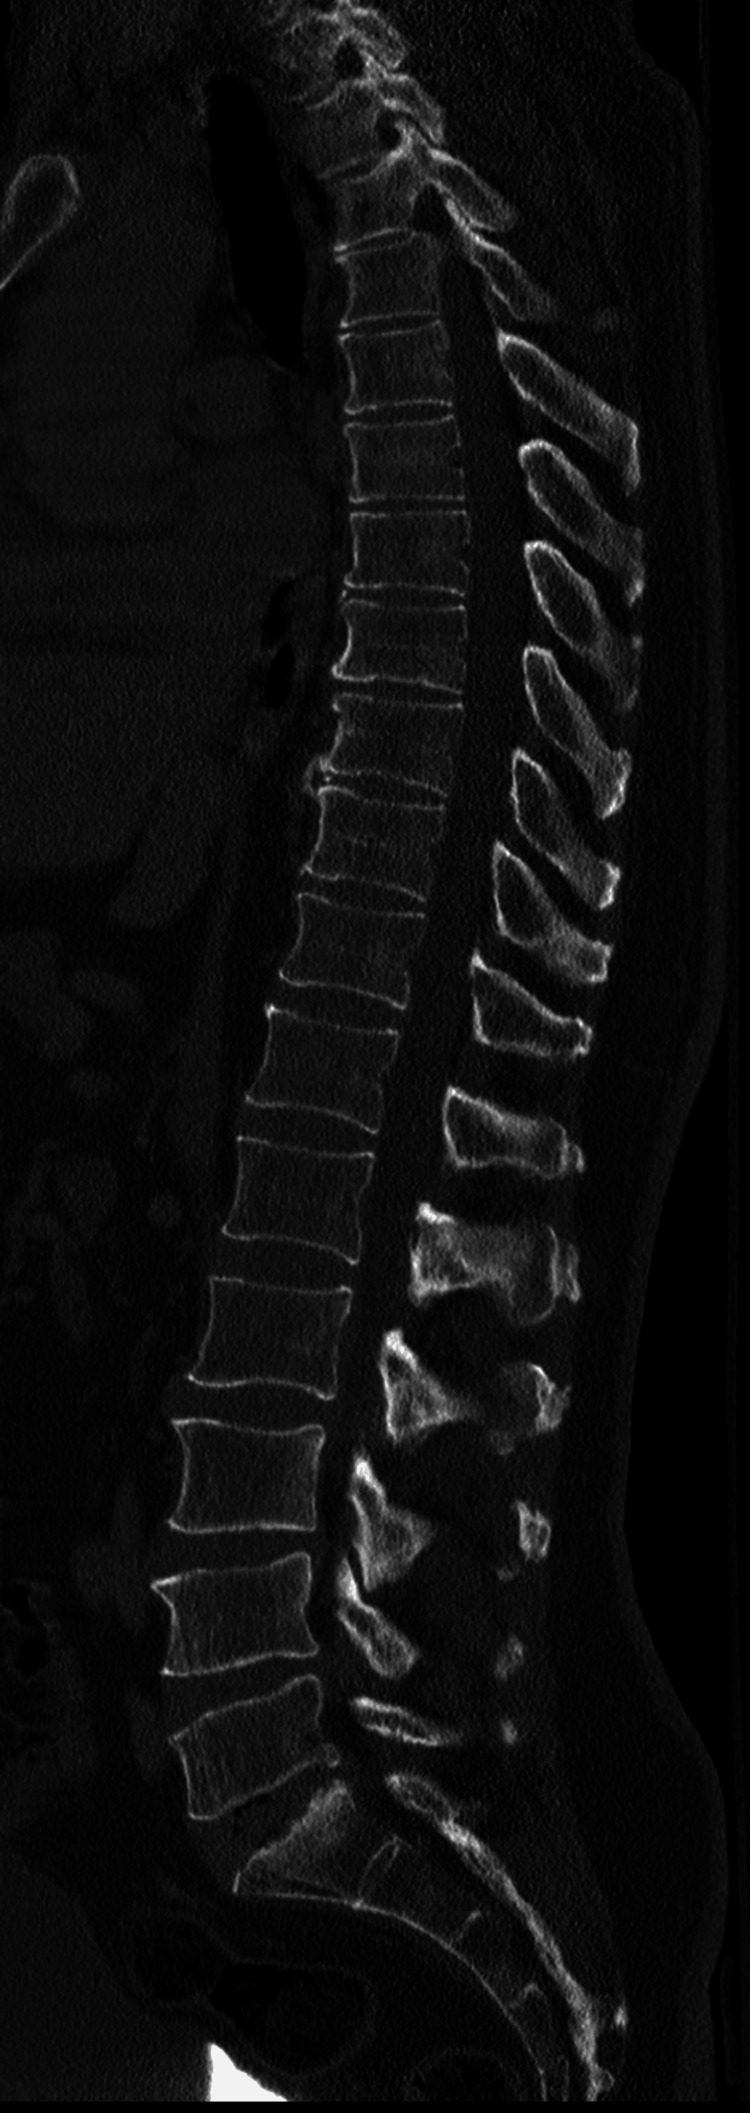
Complementary study: Dorsolumbar CT scan There were no obvious fractures of the cervical, dorsal, and lumbar vertebrae and no signs of obvious post-traumatic canal involvement. There were degenerative changes in the lumbosacral hinge.
